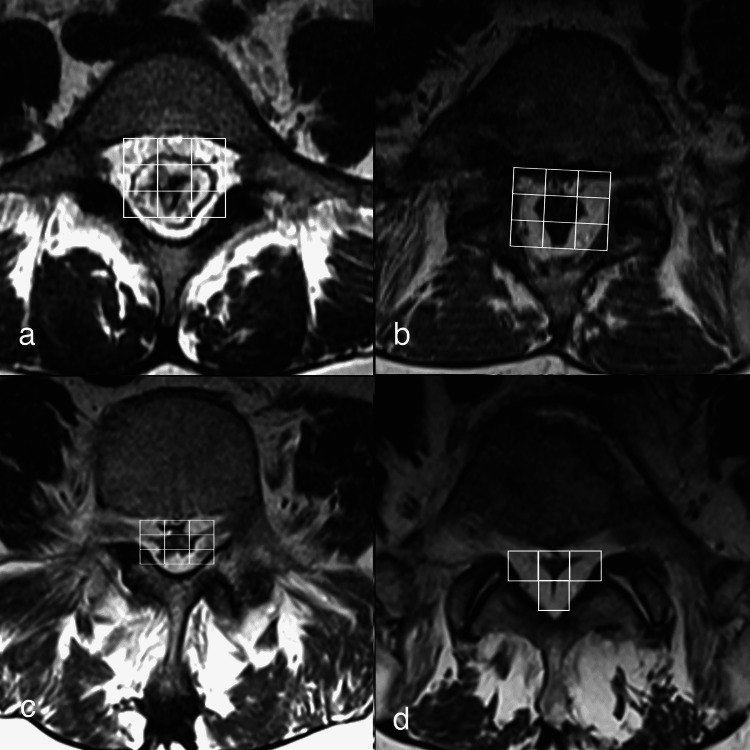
Axial T2-weighted MR images showing some practical applications of superimposed 3x3 grids and a 3+1 grid in certain overlapping grades in the Manjila classification of SEL. (a) shows mild ventral SEL (type 1). (b) shows a central (type 3) thecal sac location with moderate severity, and (c) shows central (type 3) moderate thecal sac compression. In the 3x3 grid technique, the dorsal row of squares shows varying grades of encroachment by hypertrophic facet joints/synovial cyst as shown in a-c. (d) shows severe SEL with the thecal sac restricted only to one small square, with arthritic fluid level within the facet joint, making it a subcategory Alpha (α). The MRI software function of area (cm-square) for exact measurements of the spinal canal and dural sac needs to be compared to the Manjila classification using grids as shown in the images above. SEL: spinal epidural lipomatosis Source: Authors of the current article
